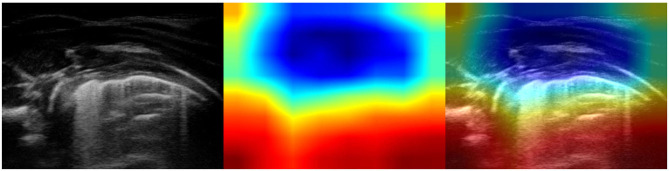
Example of lung ultrasound image of a healthy lung incorrectly classified as bronchiolitis by the best performance model (Inception-v3). (Left) Original LUS image; (Middle) Class activation mapping produced by the Grad-CAM visualization; (Right) The class activation mapping overlaid transparently on the original LUS image. Note that red and orange regions correspond to high scores for the predicted class and highlight multiple, confluent long vertical artifacts. These visual features are typical of bronchiolitis but can also be observed in very young healthy infants (~ 6 months of life). Therefore, the optimal model “mistake” results in the recognition of multiple long vertical artifacts.
